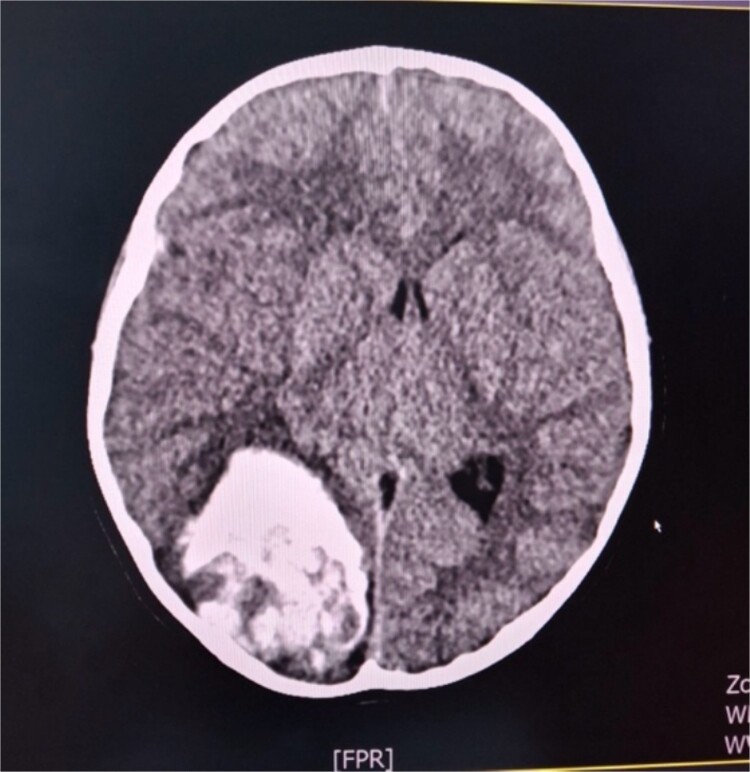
Axial cerebral CT scan shows massive hemorrhaging tumor in the right occipital region with perifocal oedema.
